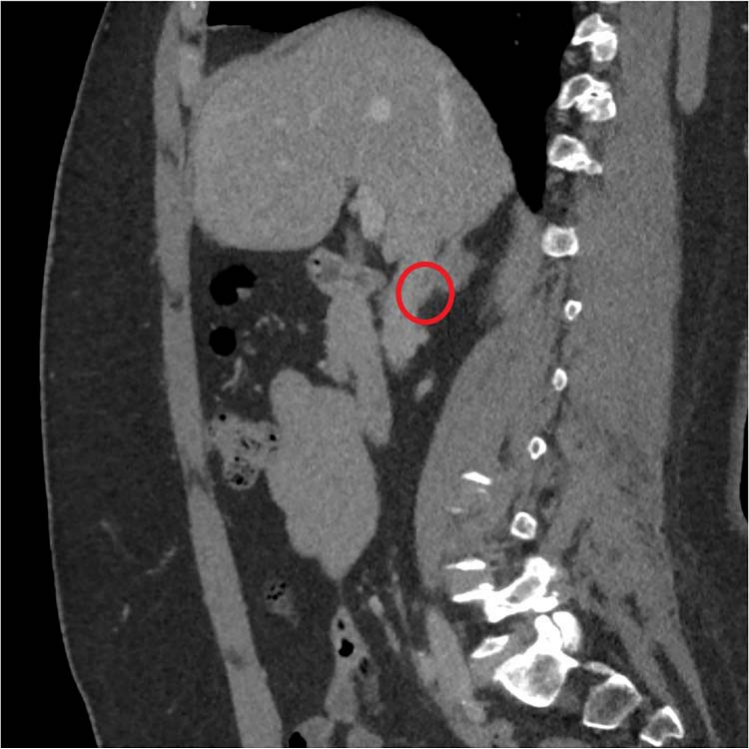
Sagittal 3D reconstruction of CT scan in venous phase. In particular, the images had raised the suspicion of the short course of the adrenal vein in the lower lateral lip of the mass.
